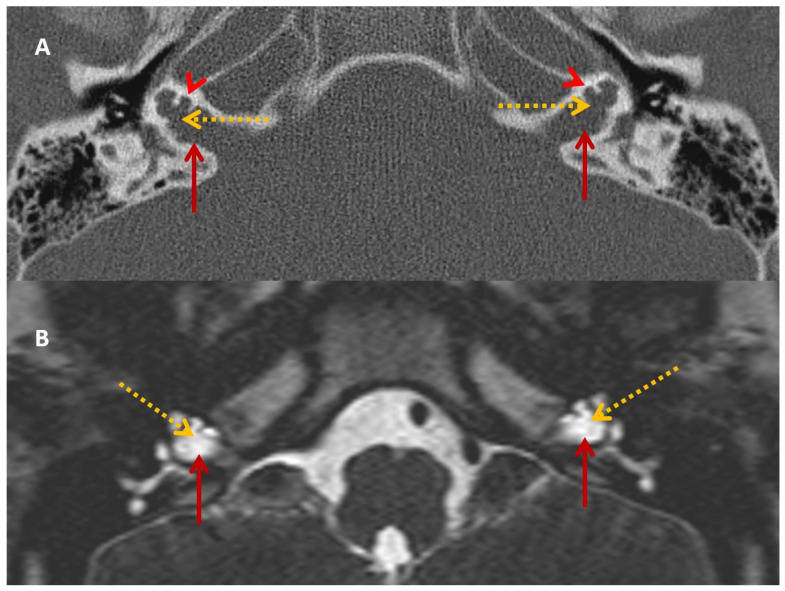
SA—HRCT and MRI. HRCT (A) and T2 Drive MRI (B) in sibling SA showing bilateral bulbous dilatation involving the fundus of the internal auditory canal (maroon arrow) and bilateral incomplete separation of the basal turn of the cochlea (red arrowhead) from the fundus of the internal acoustic canal (dotted yellow arrow), classical of X-linked gusher disorder.
